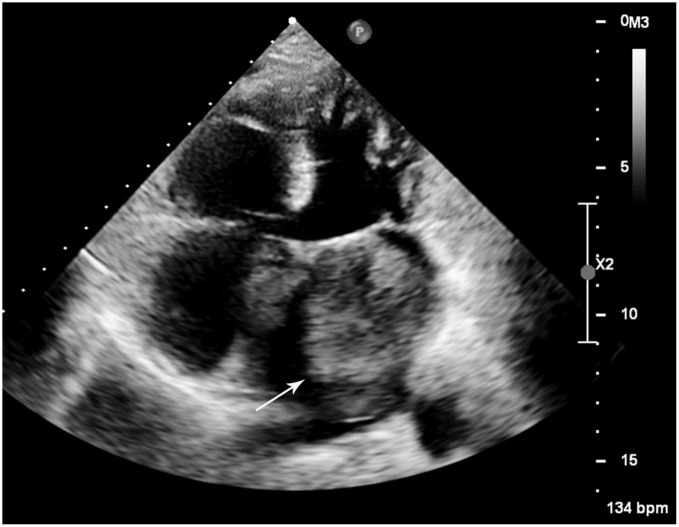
Transthoracic echocardiogram of the left mass. A large hypoechoic mass (white arrow) was revealed in the left atrium attaching to the lateral atrial wall *via* a broad base.
